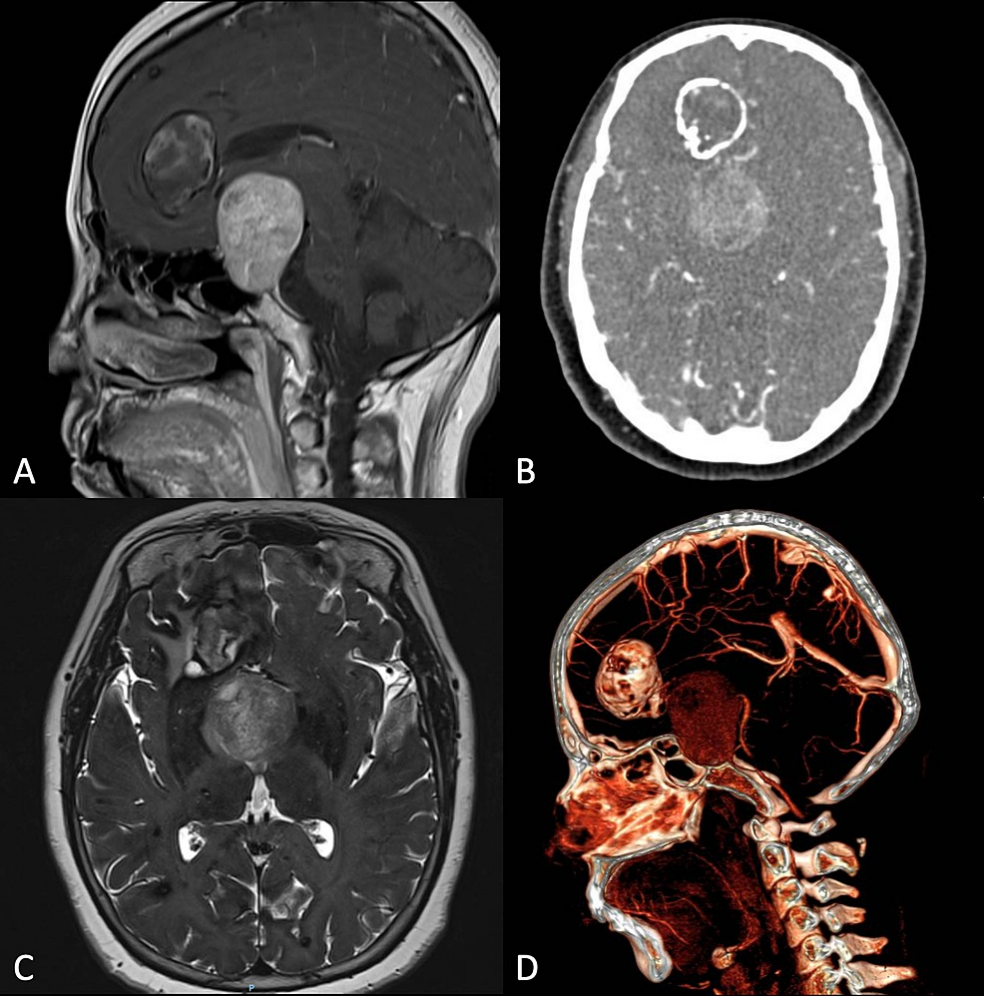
Brain MRI, CT scan, and CT angiography 3D reconstruction. A) Sagittal T2-flair brain MRI showing the sellar tumor with heterogenous intensities causing elevation of the third ventricle’s anterior floor and a calcified lesion on the frontal lobe with a hypointense core. B) Brain CT scan showing two lesions. A calcified lesion located at the right frontal lobe and a homogeneous lesion (PAs) at the sellar level. C) Axial T2-weighted brain MRI showing isointense sellar and right frontal heterogeneous lesions. D) CT angiography 3D reconstruction showing hypervascularization of both lesions.
